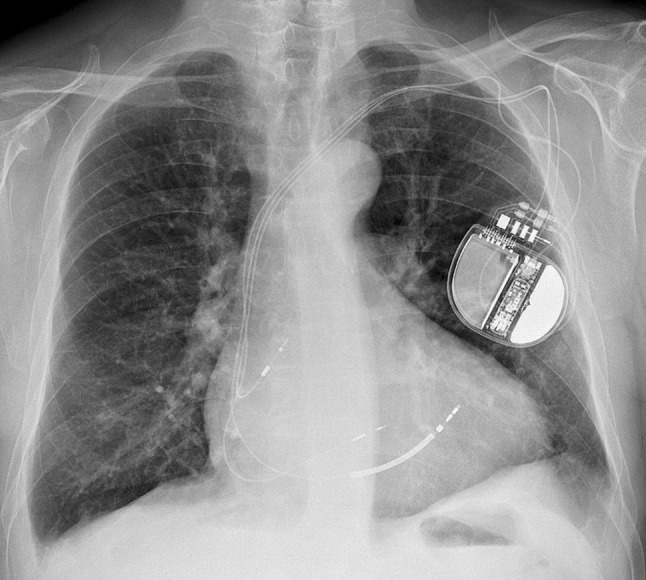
Chest radiograph in anterior-posterior projection demonstrating final position of right atrial lead, left ventricular lead and right ventricular shock lead
